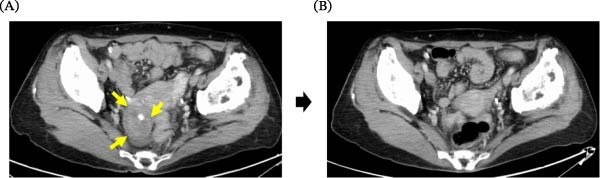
Perioperative computed tomography images of the pelvis in Case 2. (A) The findings 2 weeks before surgery. (B) The findings 2 months after surgery. The yellow arrow indicates the enlarged right ovary.
